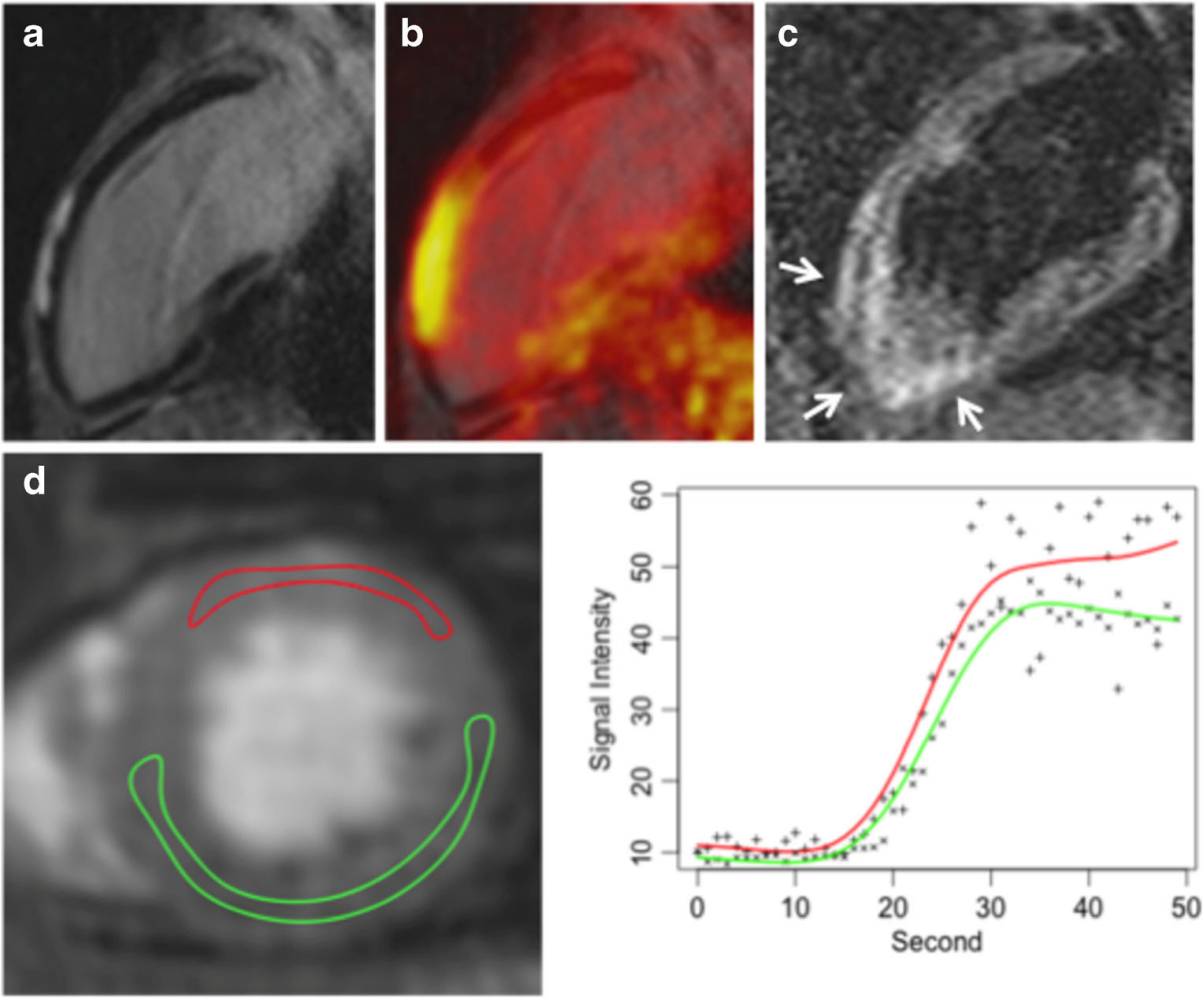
^18^F-FDG PET/MRI in a patient with acute viral myocarditis caused by parvovirus B19. (A) Late-gadolinium-enhanced MRI long-axis view demonstrating typical subepicardial enhancement in the anterior left ventricular wall that was in excellent spatial agreement with increased ^18^F-FDG uptake on fused images (B). (C) T2-weighted images revealed an oedema in the LV anterior wall. (D) Dynamic perfusion imaging revealed hyperaemia in the LV anterior wall. (With kind permission from Ref 50-Nensa, Poeppel 2014)
